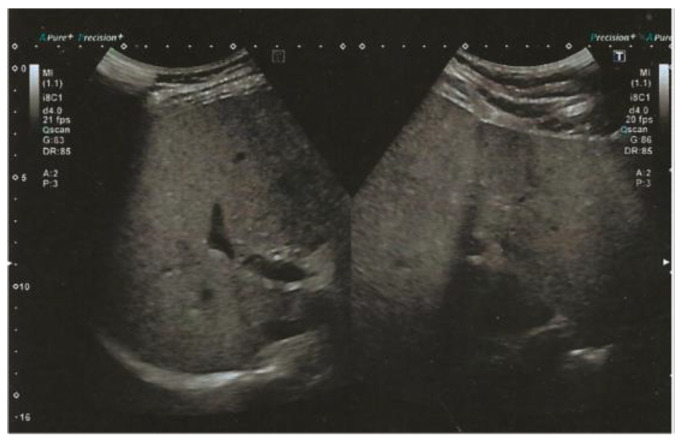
Abdominal ultrasound performed at baseline showed a diffuse increase in liver echogenicity and slight inhomogeneity, with a liver size of 16 cm, suggesting the presence of moderate liver steatosis. No additional focal liver lesions or signs of advanced liver disease are identified.
